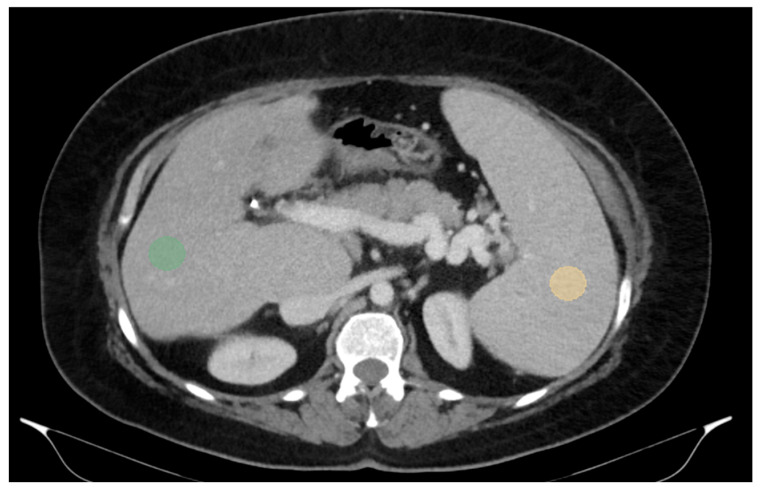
Abdominal portal-venous-phase CT scan, axial plane. Example of 2D liver and spleen segmentations. Two-dimensional liver and spleen segmentations were obtained by placing regions of interest (ROIs) in the right liver lobe at the level of the 9th right intercostal space (green ROI) and in the spleen (orange ROI), while avoiding major vascular structures.
